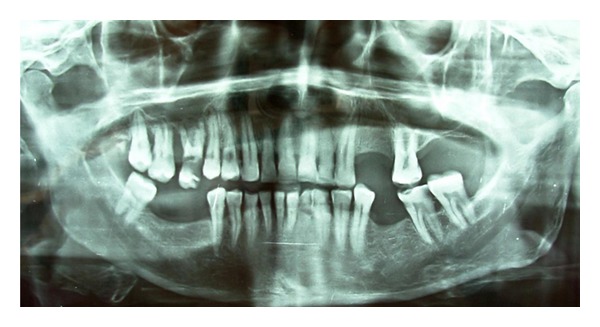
Orthopantomogram showing destruction of interdental bone between maxillary right first and second molars, disruption of the corticated border of the floor of right maxillary sinus.
